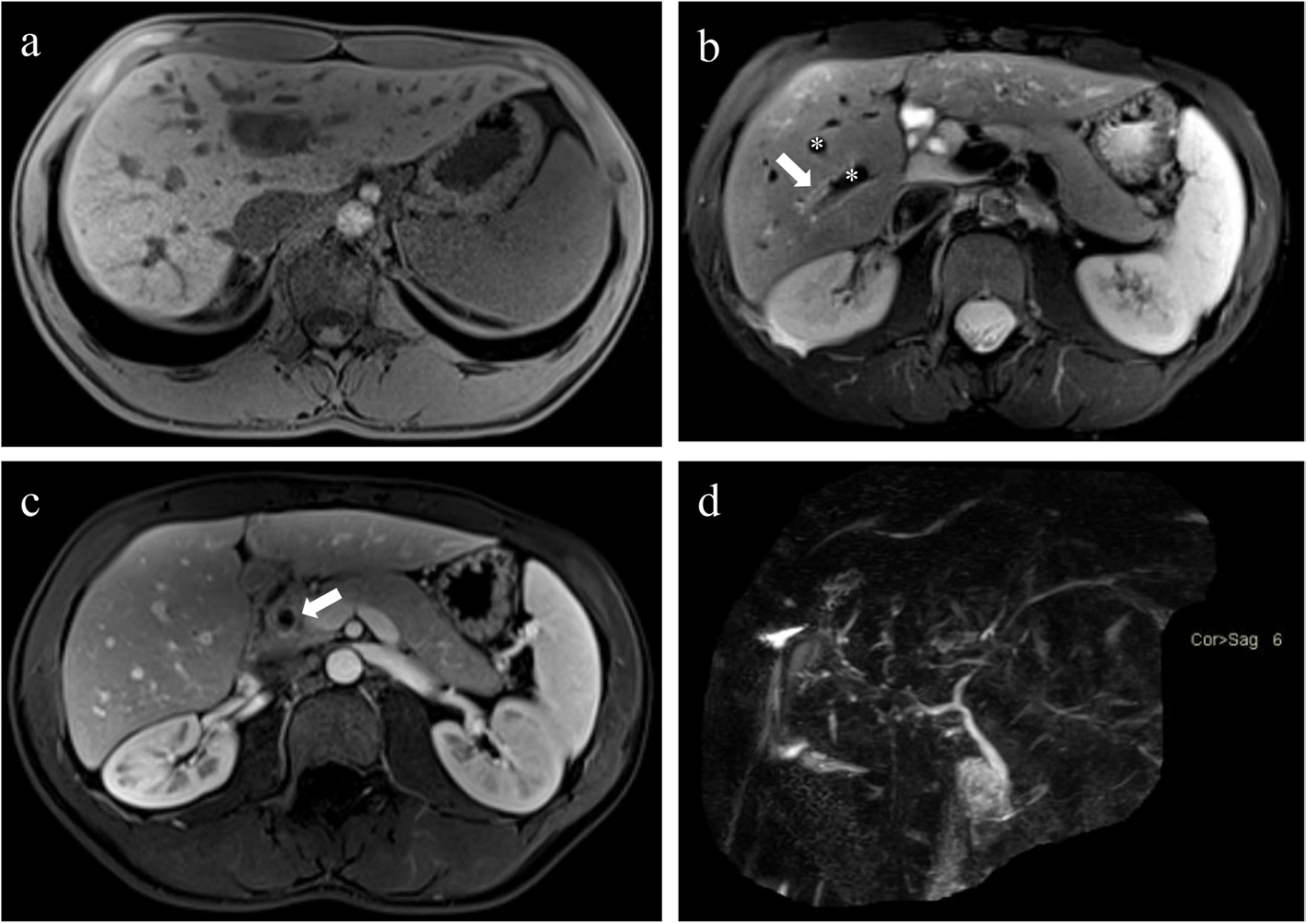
A 22-year-old male PSC patient combined with ulcerative colitis (a–d); The liver has a regular contour without nodular changes of the surface on T1WI image (a). The T2-weighted image shows mild periportal hyperintensity (arrows) and dilated intrahepatic venous ducts (*) (b). The PVP image shows the dilatation of the upper common bile duct with heterogeneous enhancement of the duct wall (arrows) (c), and the MRCP image shows a branching-tree appearance with saccular dilatations and segmental strictures of the intra- and extrahepatic biliary ducts (d)
